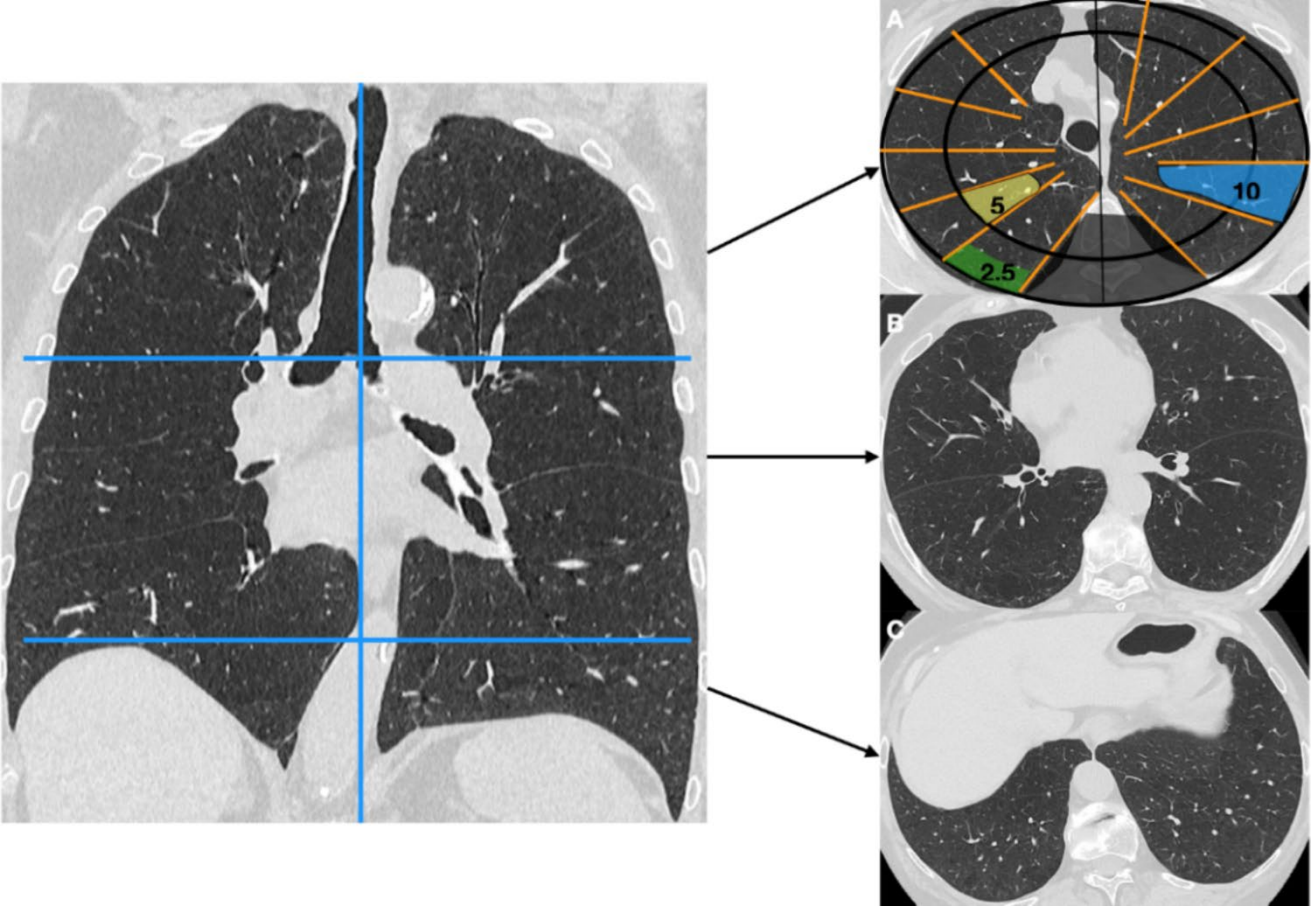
Visual score of pulmonary emphysema on computed tomography scans of three different levels of the lung (A = upper; B = middle; C = lower) are selected from high-resolution computed tomography then subdivided into smaller portions to determine the percentage of lung involvement
